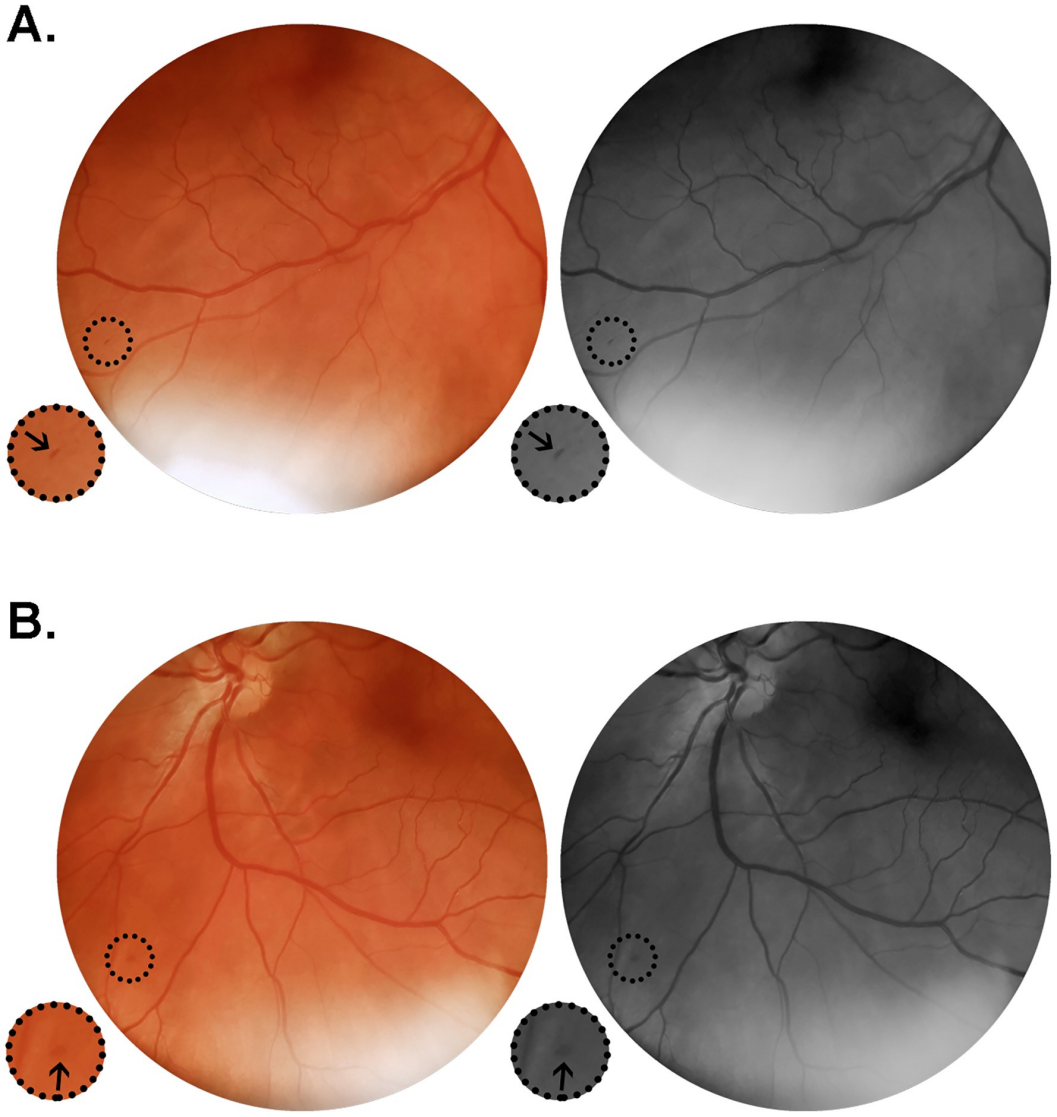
Color and red-free retinal photographs from patient #19 (G.C.X.). Images acquired on day 12 from symptoms onset, from a 49 year-old man in regular treatment for arterial hypertension, admitted on the eight day after onset of fever, cough, myalgia and progressive dyspnea. Lung CT on admission showed typical viral pneumonia findings extending through 25–50% of the parenchyma. The patient evolved to a severe phenotype of the disease. (A) Right retina presenting with isolated microhemorrhage at the periphery close to the inferior temporal vasculature. (B) Left retina showing isolated microhemorrhage at the inferior nasal retinal quadrant. Insets represent delimited areas with 2x magnification.
